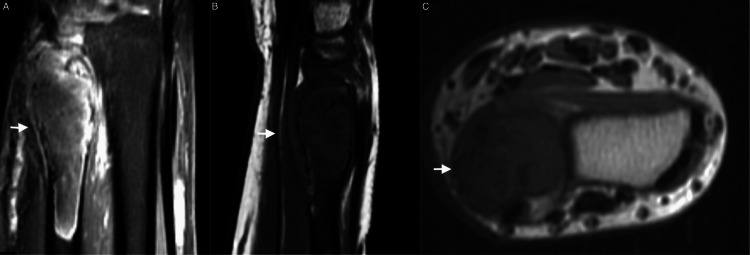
T2 coronal (A) and T1 sagittal (B) and axial (C) MRI showing an expansile lesion centered at the distal ulna with cortical thinning and minimal bone marrow edema
